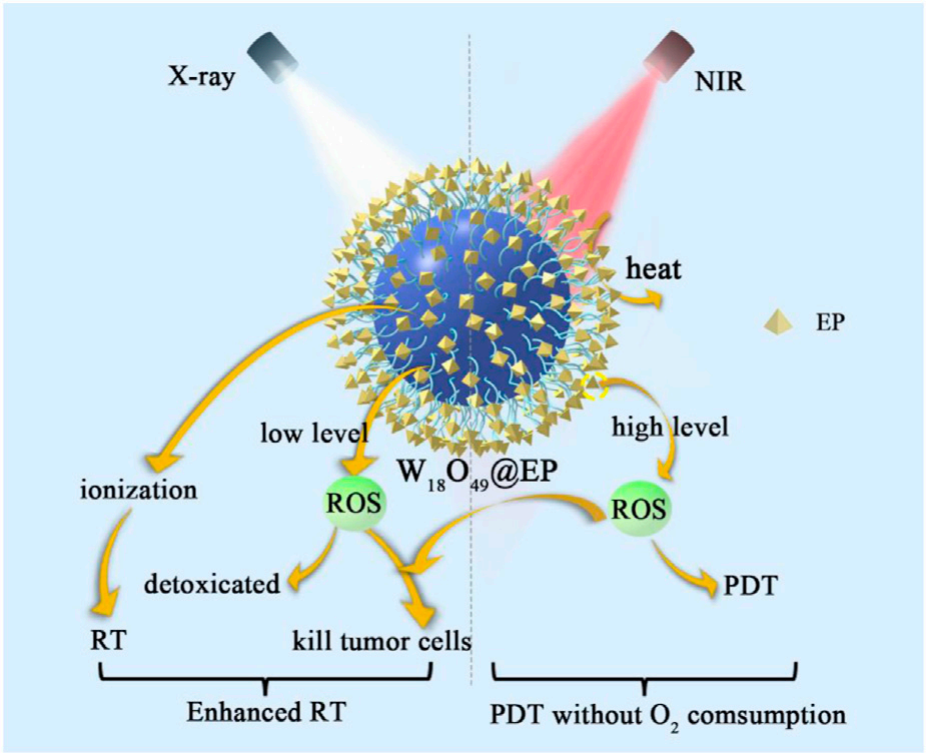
The mechanism of W_18_O_49_@EP under NIR and X-ray.
